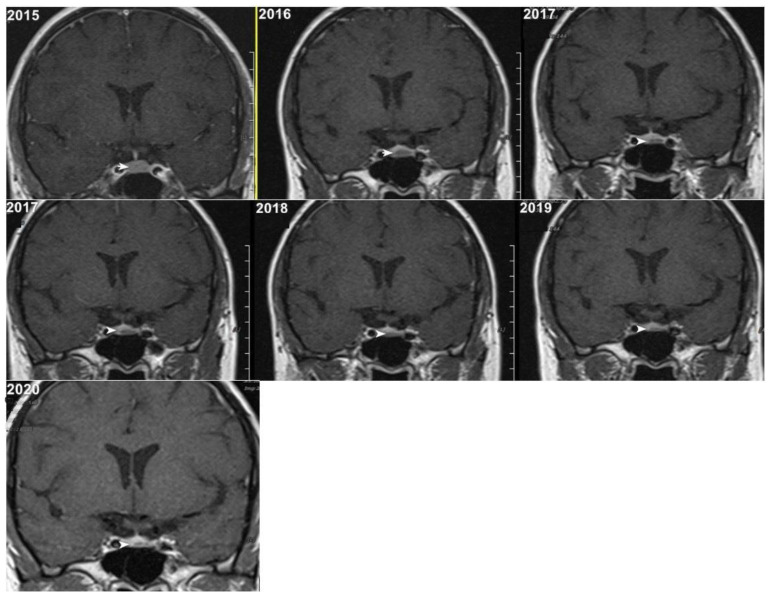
Representative coronal images (2015–2020) of a stable microadenoma (arrows) of a 33 Y/O woman. The left upper image from 2015 is a delayed image, and the other six images are dynamic MRI follow-ups for this patient. In 2017, the patient had two follow-ups in the first and second half of the year. In the 5 years of follow-up, no size change can be seen.
